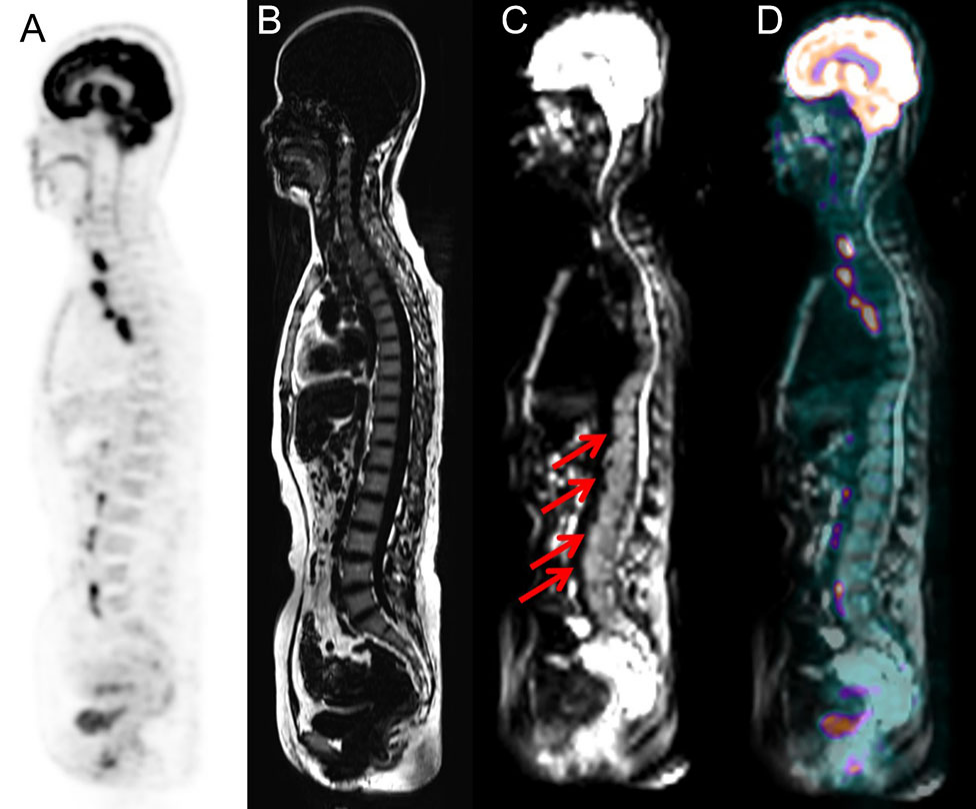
Images of a 51-year-old woman with small lymphocytic lymphoma. BMI was detected via PET/MRI due to the presence of intense signal on the MRI (arrows), while PET did not show heterogeneously high FDG uptake. The presence of BMI was confirmed by routine staging BMB, which was performed at the level of the posterior iliac crest. (A) PET image derived from PET/MRI. (B) TIWI image derived from integrated PET/MRI. (C) DWI image derived from integrated PET/MRI. (D) Fused whole-body integrated PET/MRI.
